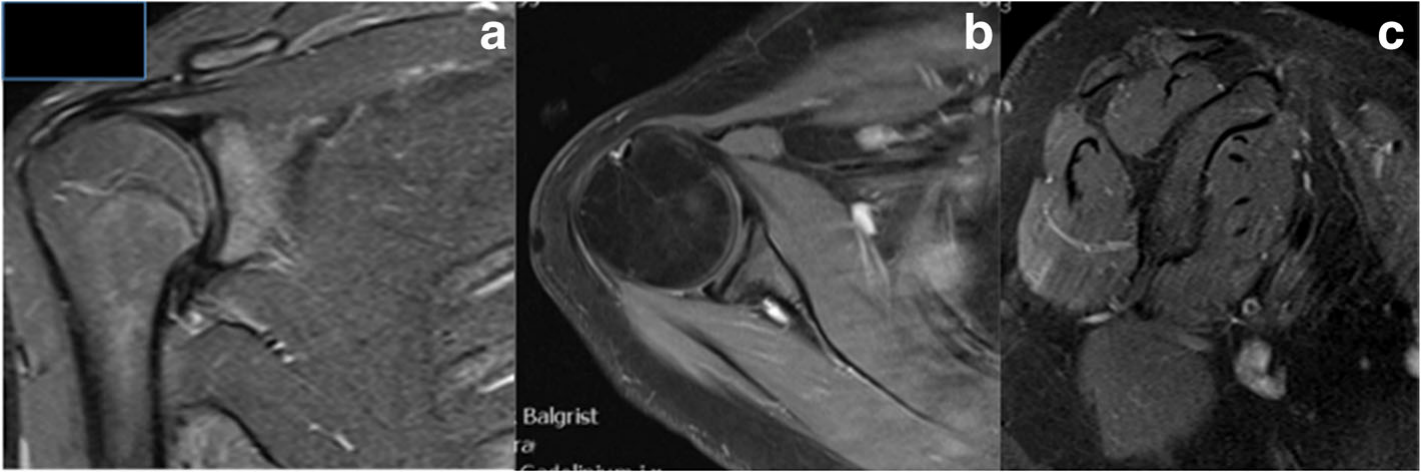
Magnetic resonance imaging (Fig. 4a frontal plane; Fig. 4b axial plane; Fig. 4c parasagittal plane) of the patient’s shoulder at 11 years of follow-up shows normal rotator cuff muscles without atrophy and fatty streaks, but the absence of the deltoid muscle
